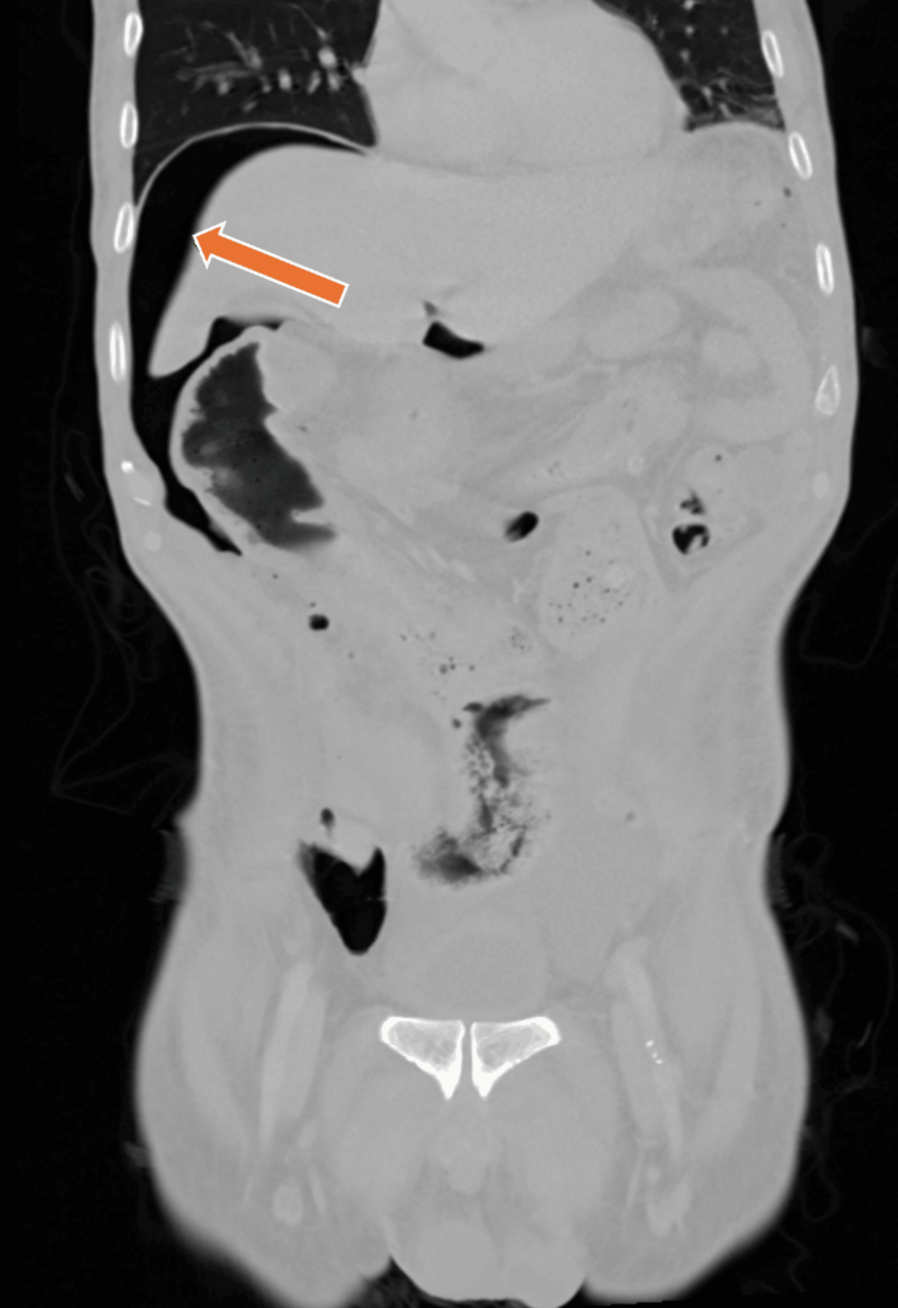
Repeat contrast-enhanced CT of the abdomen and pelvis demonstrating pneumoperitoneum (arrow) with free intraperitoneal air beneath the diaphragm, consistent with bowel perforation and prompting emergent surgical intervention.
